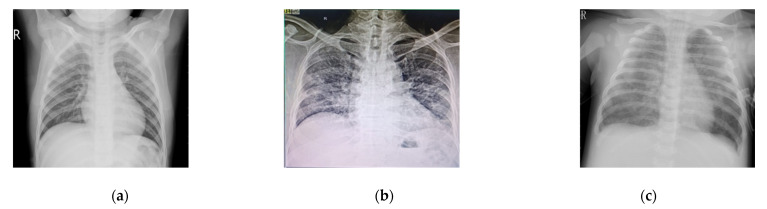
X-ray of (a) normal patient with no COVID-19 symptoms for pelvis surgery with negative PCR test; (b) is a patient with COVID-19 symptoms, positive PCR test on day 1 and 3; (c) is a pneumonia patient with negative PCR test on day 1, 3 and 5.
